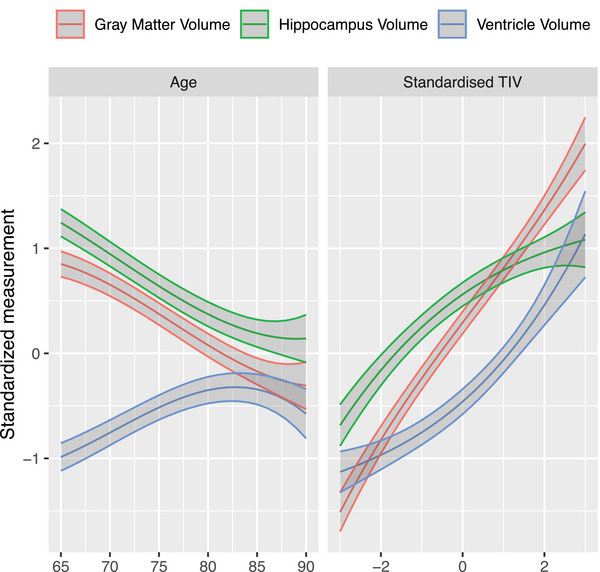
Nonlinear trajectories of MRI measures with 95% confidence intervals by age and standardized TIV in combined cohorts of ADNI and OSTPRE. ADNI, Alzheimer's Disease Neuroimaging Initiative; OSTPRE, The Kuopio Osteoporosis Risk Factor and Prevention Study; MRI, magnetic resonance imaging; TIV, total intracranial volume.
